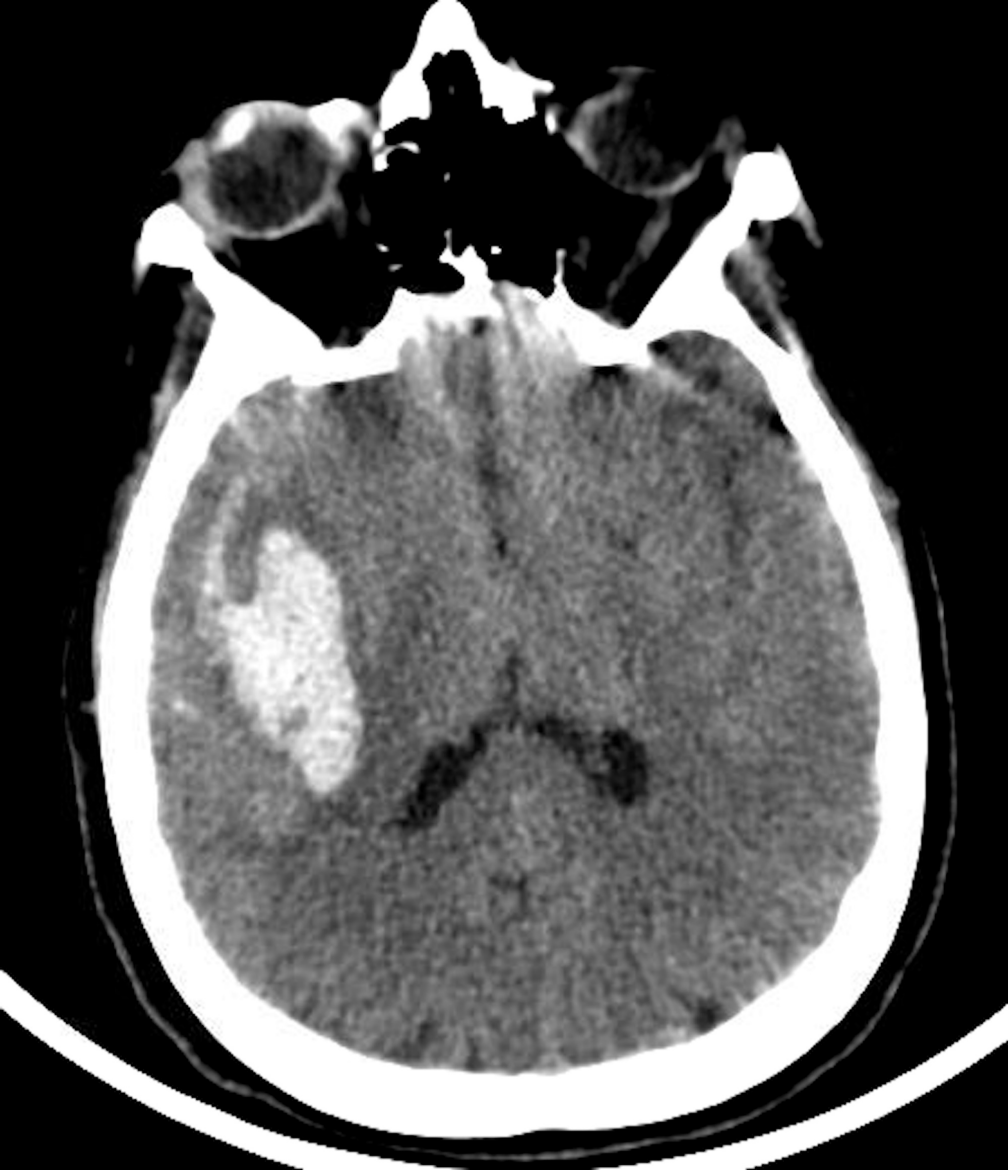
Initial non-contrast head computed tomography (CT) demonstrates right temporal lobe intracerebral hemorrhage with subarachnoid hemorrhage within the sylvian fissure.
